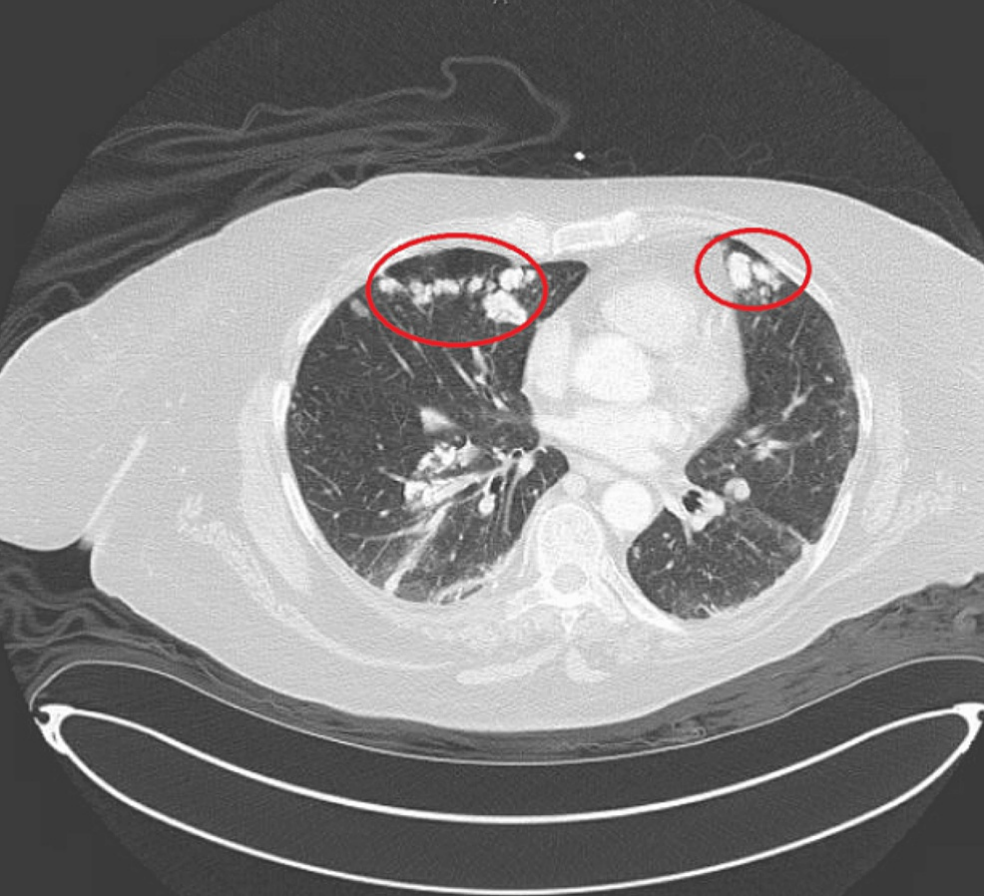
CT chest scan from April 2022 considered the potential for pulmonary metastatic calcifications situated in the anterior part of the left and the right lung (red circles), potentially from a recurrent right pelvic chondrosarcoma. Importantly, the size of the nodules has increased from the previous CT scan.
